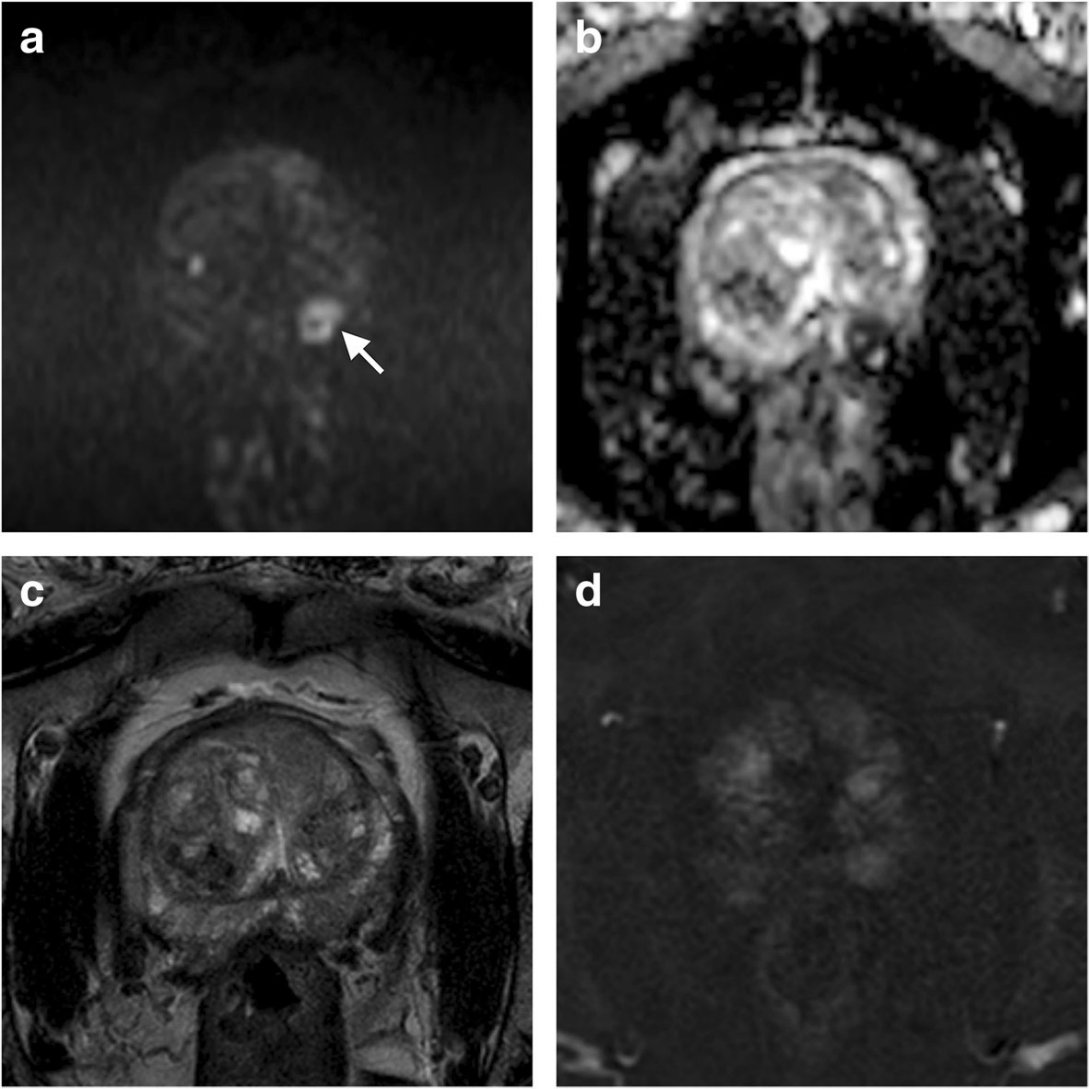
Application of the Prostate Imaging Reporting and Data System (PI-RADS) version 2 in interpreting a finding in the peripheral zone (PZ) of a biopsy-naïve 62-year-old man undergoing prostate multiparametric magnetic resonance imaging (mpMRI) for elevated prostate specific antigen (PSA) level. The dominant sequence, *i.e.*, a transverse diffusion-weighted imaging (DWI) sequence, showed an area (< 15 mm in size) of restricted water diffusion in the left midgland PZ, as testified by high signal intensity on the *b* = 2000 s/mm^2^ image (arrow in a) and corresponding marked hypointensity on the apparent diffusion coefficient (ADC) map (b). This finding was classified as PI-RADS 4 accordingly, with additional ancillary suspicious features such as hypointensity on transverse T2-weighted imaging (c) and focal early contrast enhancement on subtracted T1-weighted imaging (d). Pathology after radical prostatectomy found a Gleason score 7 (3 + 4) T3a N0 cancer
